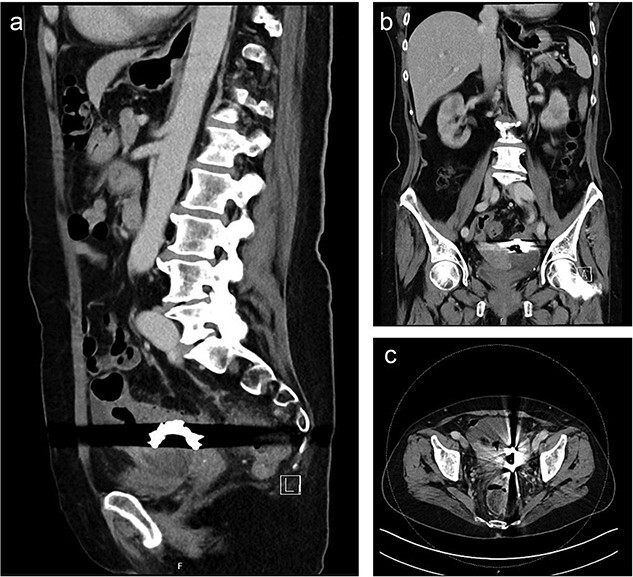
Sagittal (a), coronal (b) and axial slice (c) of a preoperative CT scan showing the ingested denture in the sigmoid colon with adjacent fluid collection and vesical air inclusions.
